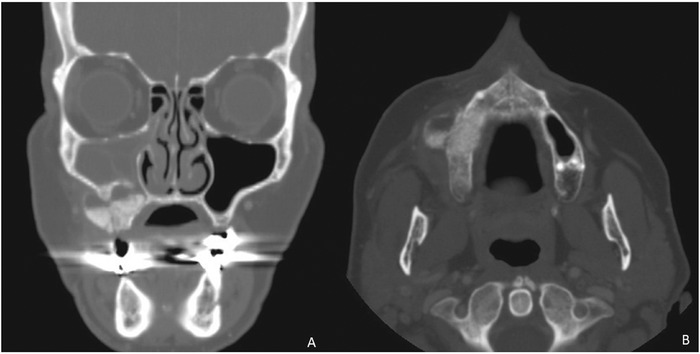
(A) Coronal and (B) axial computed tomography scan of a patient who had migration of the sinus lift graft material into the soft tissues of the cheek, resulting in extensive facial cellulitis and requiring debridement.
